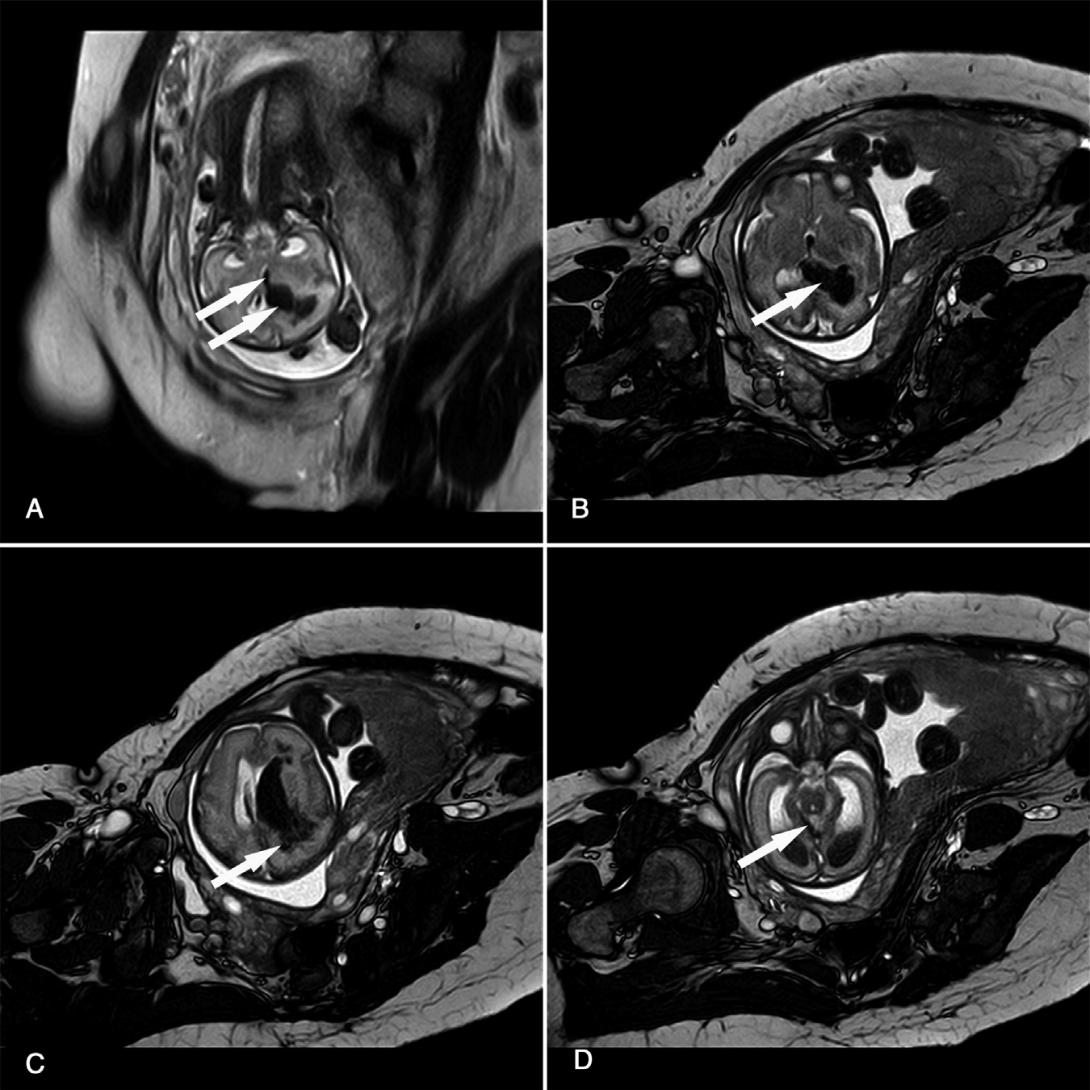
Intrauterine T2-weighted magnetic resonance images (MRI) demonstrating massive and multifocal hemorrhagic lesions (arrows) (A) the third ventricle and bilateral ventricle (B) bilateral ventriculomegaly (C) the right frontal and parietal lobes (D) tentorial subdural hemorrhage.
